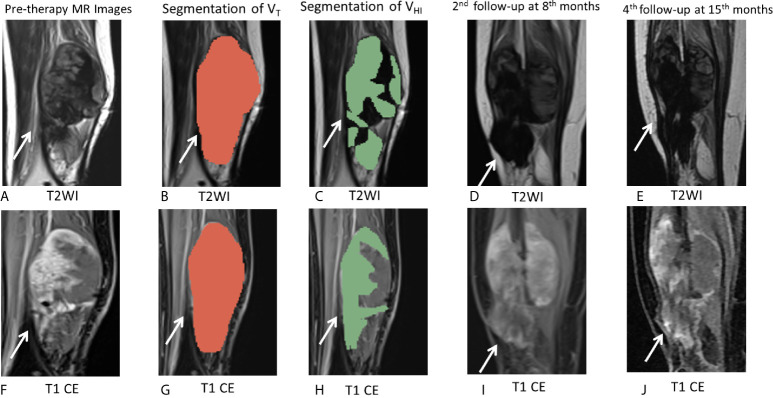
MR images of the representative cases. (A–J) A 26-year-old female patient with DF of right calf muscle with imatinib treatment of (arrows). Pre-therapy MR images refer to examination before imatinib treatment. Pre-therapy (A, F) diameter, 113.0 mm; On T2WI, V_T_ = 162,695 mm^3^, V_HI_ = 97,617 mm^3^, HI = 60% (grade = 2); On T1 CE, V_T_ = 163,750 mm^3^, V_HI_ = 106,438 mm^3^, HI = 65% (grade = 2). Segmentation of V_T_ (B, G, red zone) and segmentation of V_HI_ (C, H, green zone). Second follow-up at 8 months since imatinib treatment (D, I) diameter, 112.0 mm indicate a 0.9% decrease in tumor size. The tumor response assessment is SD, according to RECIST1.1. Fourth follow-up at 15 months since imatinib treatment (E, J) diameter, 105.0 mm indicate a 7.1% decrease in tumor size as well as a decrease in T2 and T1 CE signal intensity. The tumor response assessment is SD, according to RECIST1.1. During 15 months of follow-up during imatinib treatment, there was no evidence of progression.
